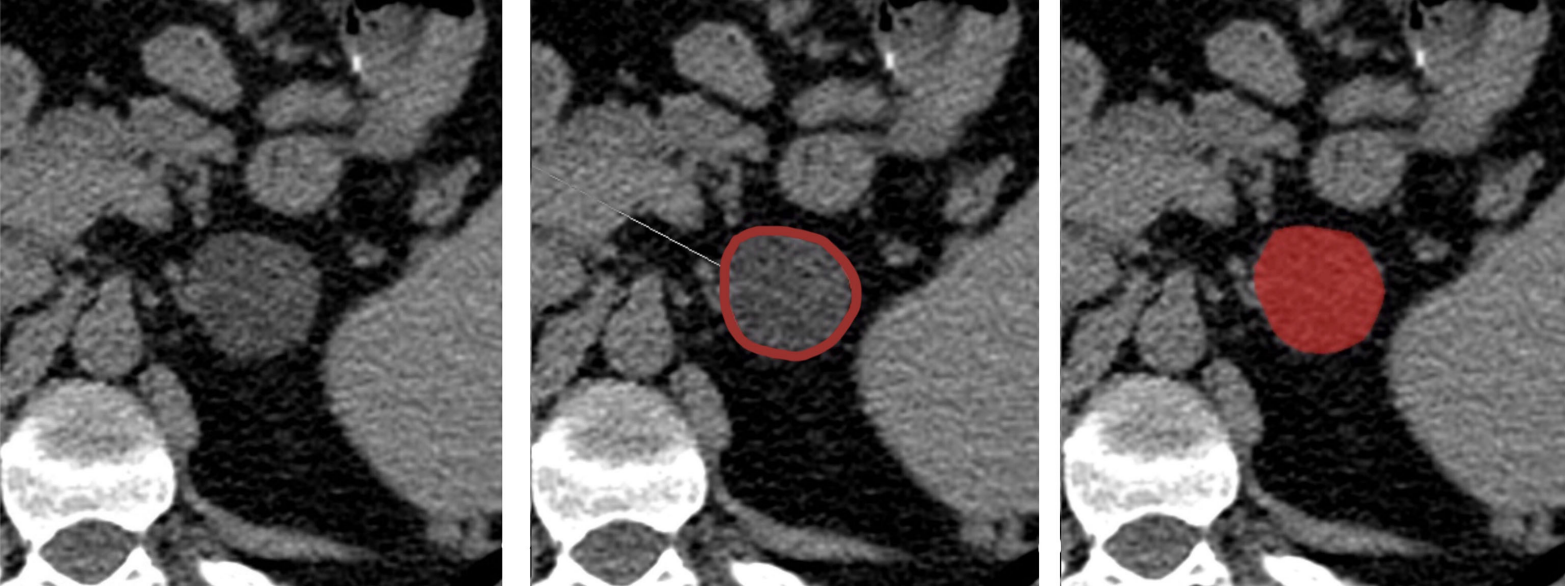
CT ROI segmentation within left adrenal lesion.
